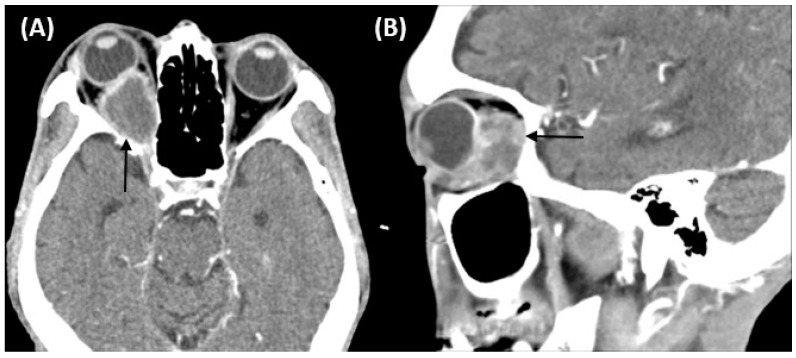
Computed tomography of the orbit demonstrating a soft tissue lesion measuring 3.3 × 2 × 2 cm in the right orbital fossa with peripheral enhancement (black arrow). The right globe and optic nerve are compressed, causing exophthalmos. (A) Axial image. (B) Sagittal image.
